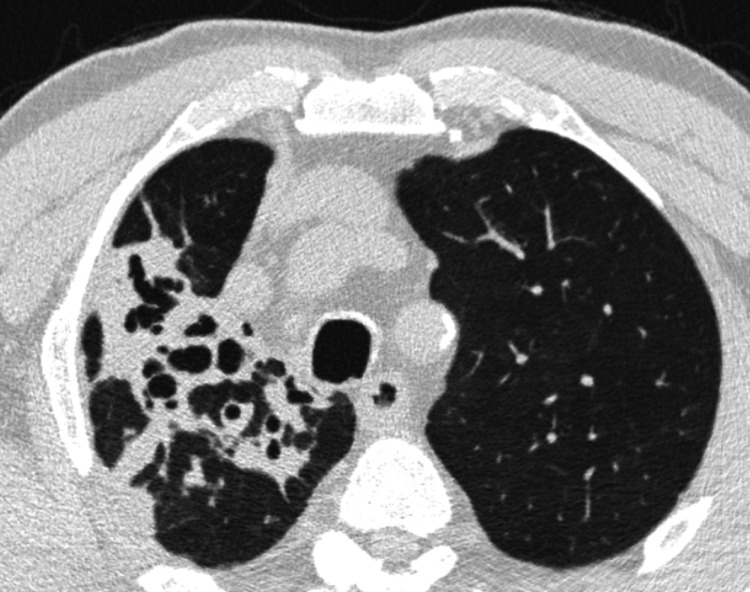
Initial Chest Computed Tomography at Presentation
